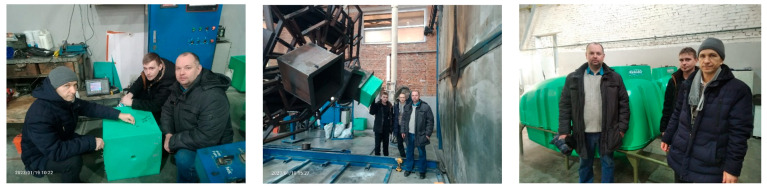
Testing of the proposed ultrasonic inspection method on the AVAGRO LLP facility.
